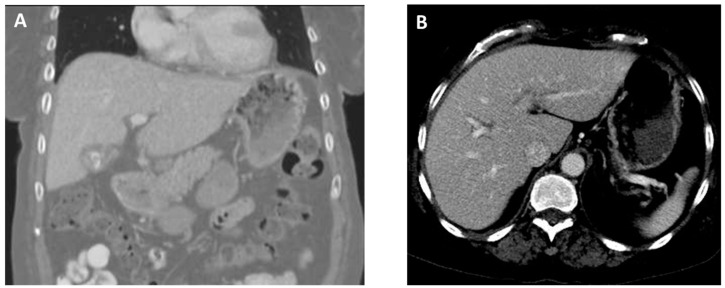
CT abdomen/pelvis with intravenous contrast obtained four months following hospital discharge shows resolution of the empyema ((A), coronal view) and perihepatic abscess ((B), axial view).
